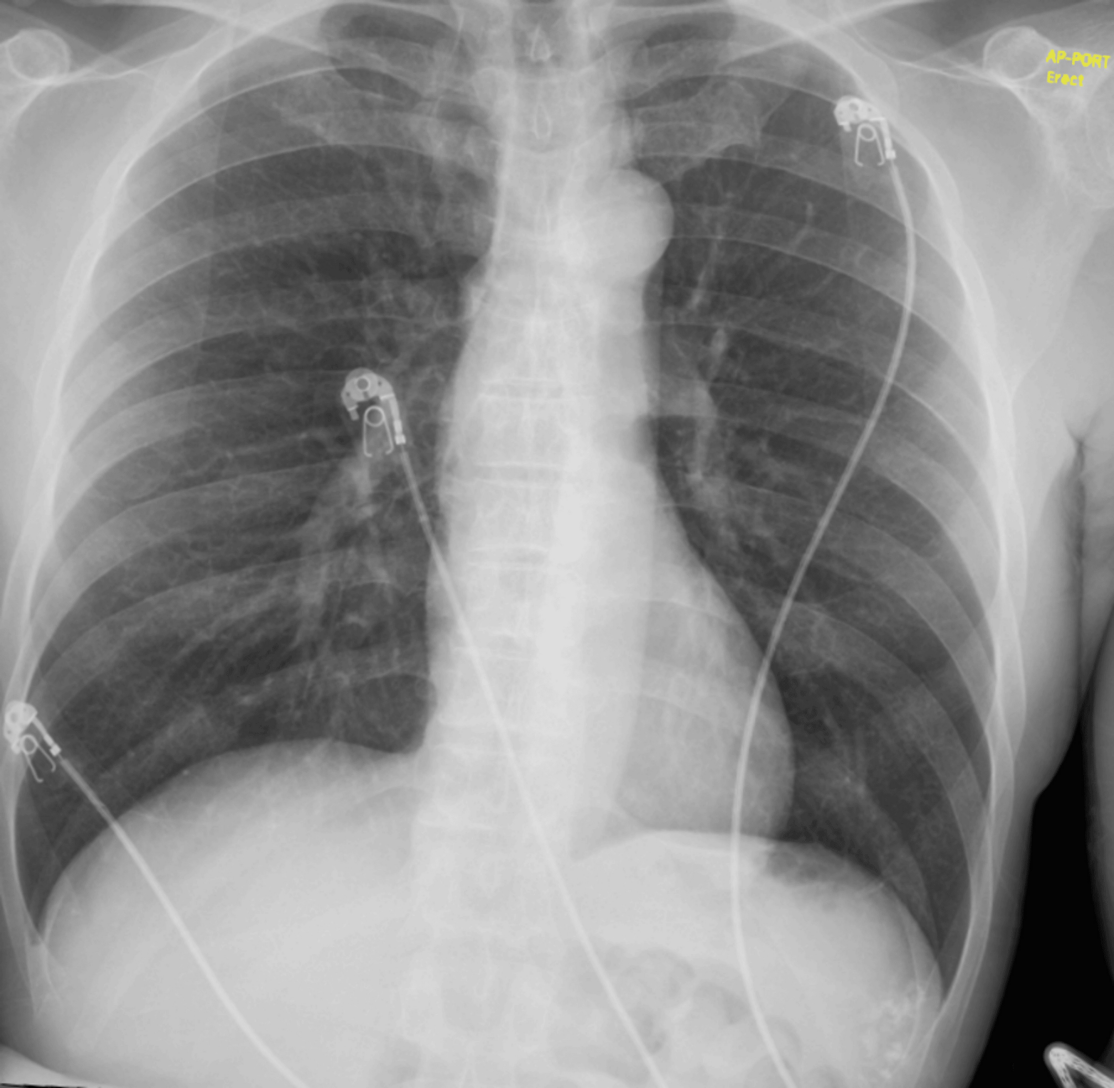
Normal chest radiograph obtained during admission Chest radiograph obtained on admission was unremarkable, with no acute cardiopulmonary abnormalities.
